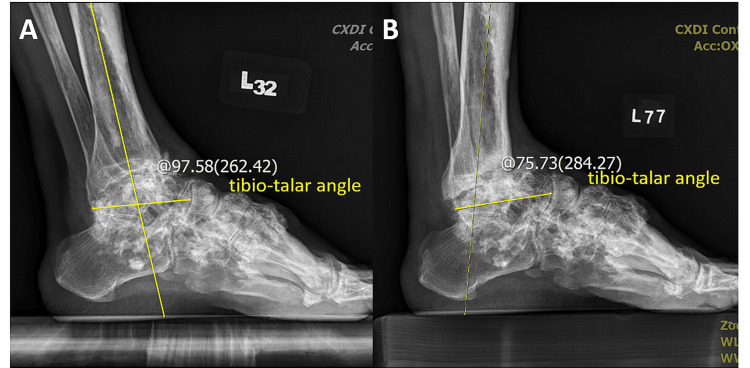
Lateral view of weight-bearing ankle radiographs. Preoperative (A) and postoperative (B) images. The tibio-talar angle was corrected from 97.58° to 75.73°.
